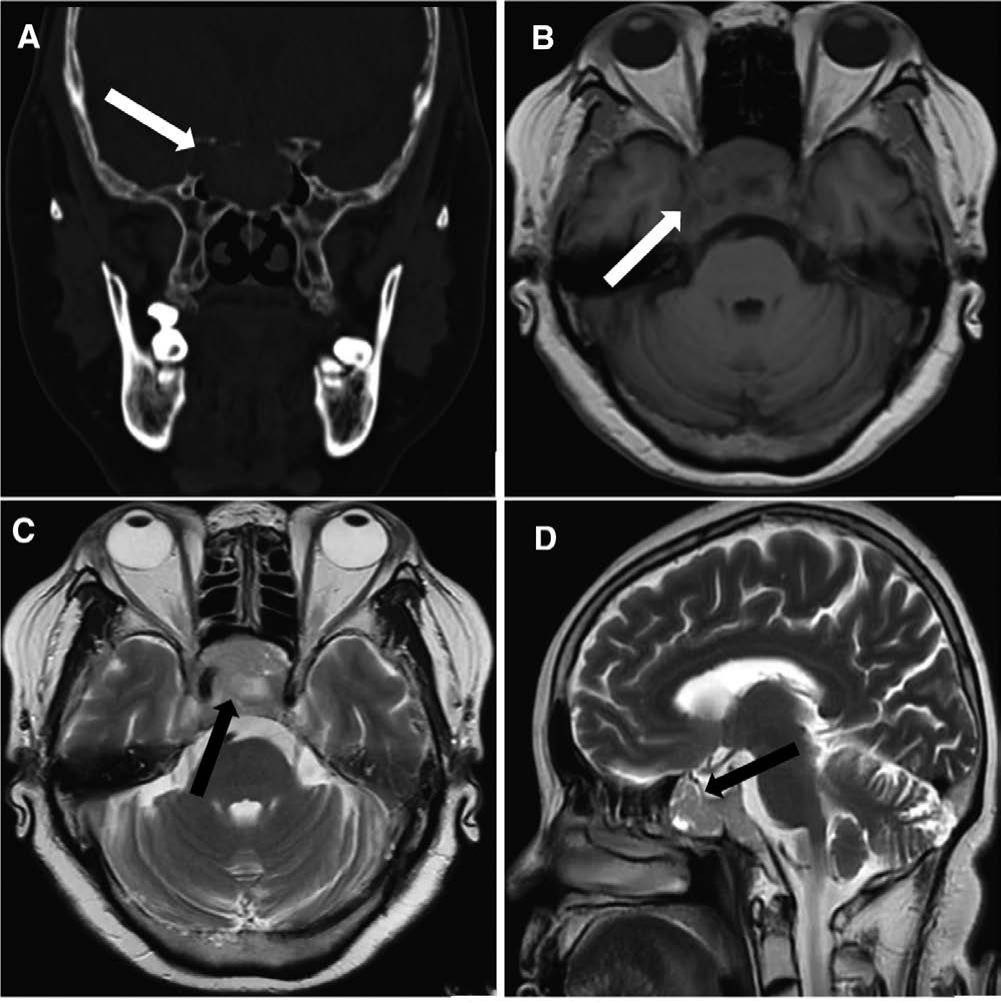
Radiological findings. (A) CT demonstrated bone destruction and a soft tissue mass on the occipital clivus (white arrow). (B) Axial view of the MR T1 revealed irregular isointense signal in the sphenoid sinus and saddle area (white arrow). (C and D) Axial view and sagittal view of the MR T2 showed isointense signal/slightly high signal in the sphenoid sinus and saddle area (black arrow). CT = computed tomography.
